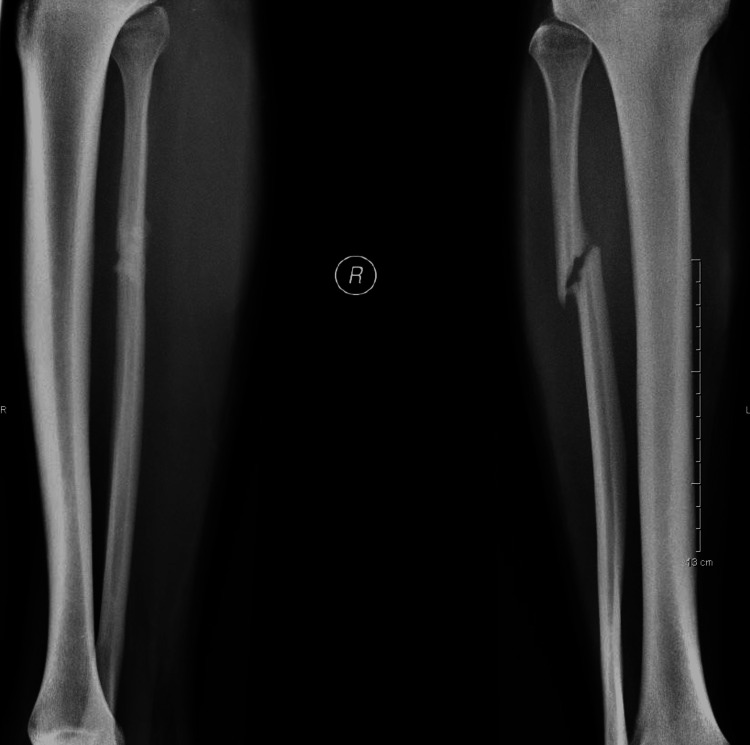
Initial X-rays where the hypertrophic non-union of the fibula can be observed
